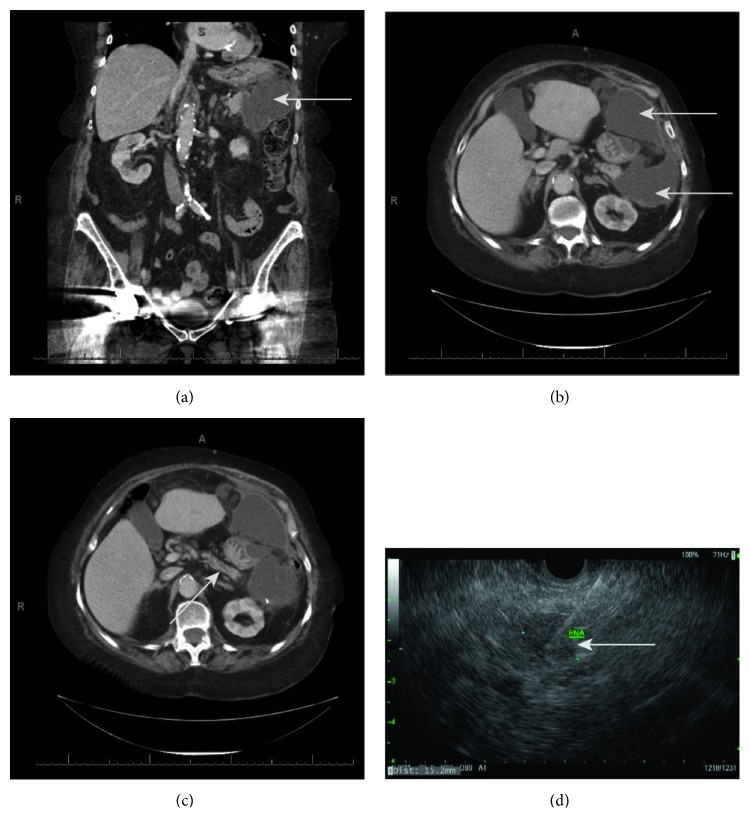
(a) Coronal section from initial CT showing large fluid collection (gray arrow) adjacent to the tail of the pancreas. (b) Axial section from initial CT showing anterior and posterior aspects of large fluid collection (gray arrows) surrounding the stomach and adjacent to the tail of the pancreas. (c) Axial section from initial CT showing mildly dilated pancreatic duct (gray arrow). (d) Still frame from EUS showing FNA needle within the visualized pancreatic mass (gray arrow).
